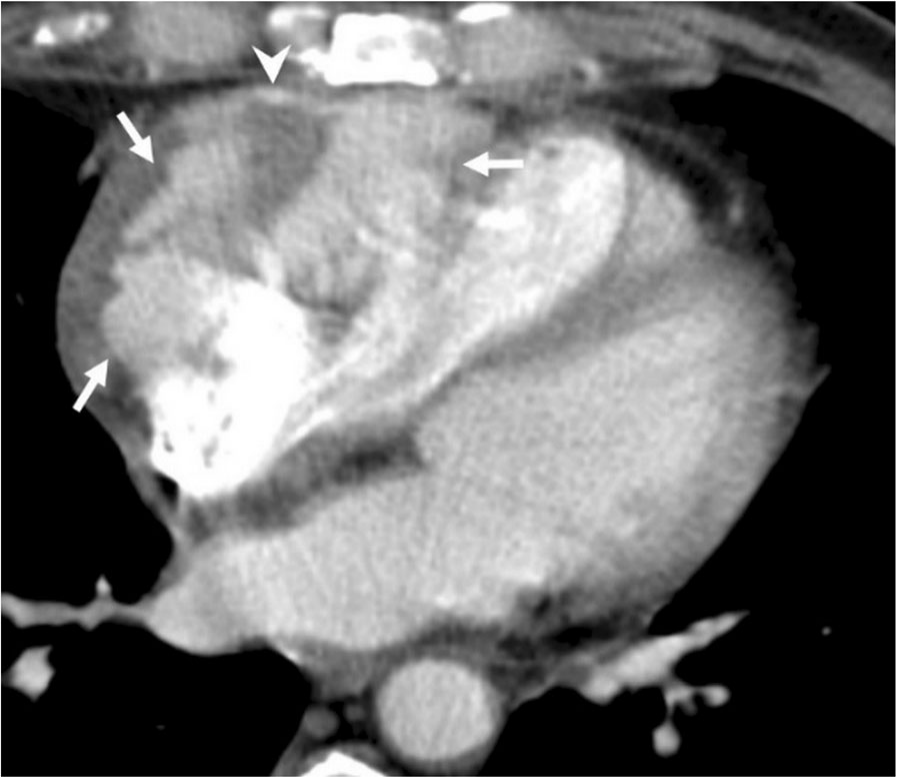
A 59-year-old man with pathologically proven primary cardiac angiosarcoma. Axial contrast-enhanced CT image reveals a highly vascular mass (arrows) invading the right atrioventricular groove, right atrium, and ventricle. Pericardial involvement (arrowhead) is also noted
